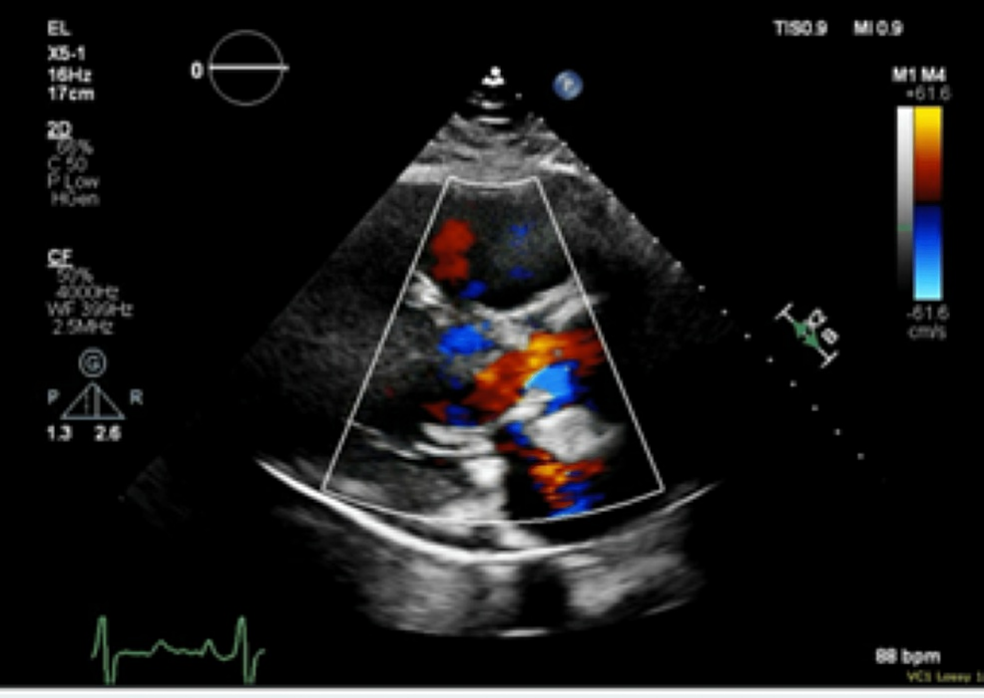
Snapshot image from the immediate TTE TTE: Transthoracic echocardiogram
